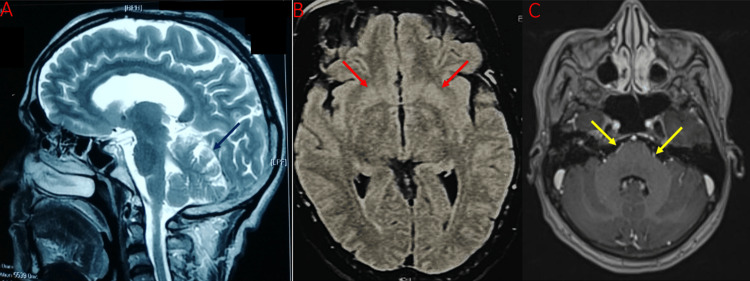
A. Brain MRI (T2 sagittal section) shows diffuse atrophy, predominantly in the olivo-ponto-cerebellar region, as observed in the fourth patient case (blue arrow). B. Brain MRI (T2 FLAIR coronal section) showing bilateral hyperintense signals in the striatum in the fifth patient case (red arrows). C. Brain MRI (CISS sequence) showing leptomeningeal and VI cranial nerve involvement in the sixth patient case (yellow arrows). CISS, constructive interference in steady state; FLAIR, fluid-attenuated inversion recovery
